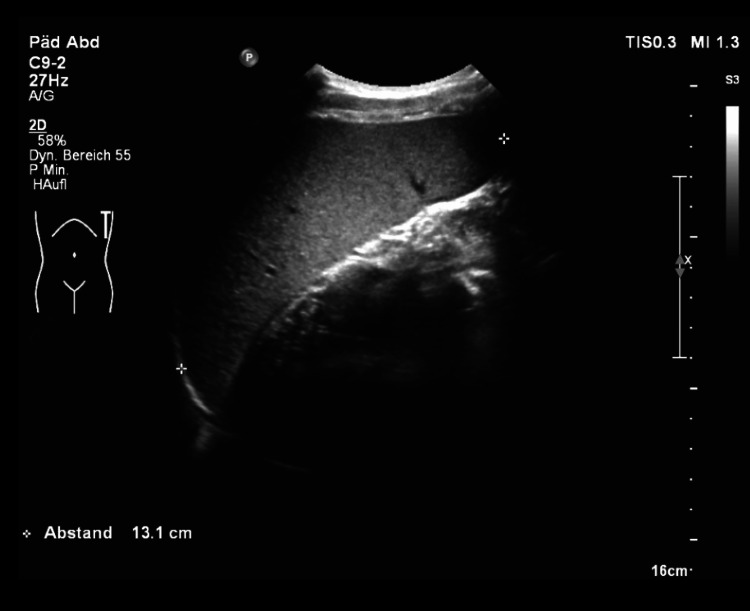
Ultrasonography of the Abdomen Showing a Slightly Enlarged Spleen Before maralixibat treatment, the spleen measured 13.1 cm (normal age-related value, 9.5–12.5 cm).
